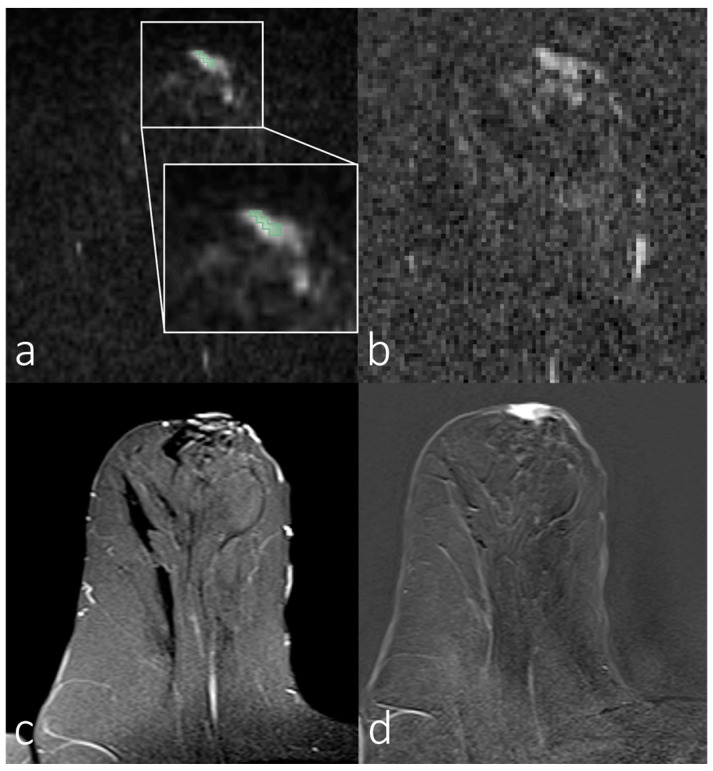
Breast magnetic resonance imaging of a woman with Paget’s disease of the nipple. (a) Manual segmentation overlaid on the diffusion-weighted image (b = 800 s/mm^2^), with segmentation highlighted in green; (b) apparent diffusion coefficient map; (c) T_2_-weighted image with fat saturation; (d) T_1_-weighted subtraction image after contrast administration.
